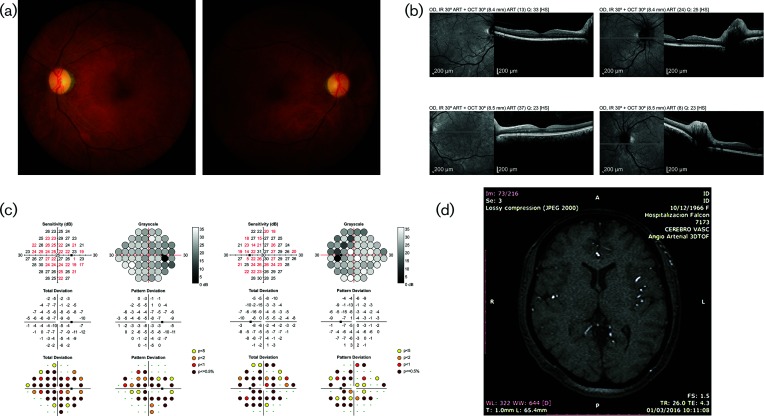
Patient described in Case 1. (a) Fundus photographs showing the optic nerve heads and maculas of the left and right eyes. (b) Optical coherence tomography (OCT) of the left and right eyes showing optic disc swelling; both macular scans are normal. (c) Visual field testing results showing diffuse contrast sensitivity loss. (d) Magnetic resonance imaging (MRI) of the brain showing no abnormalities.
